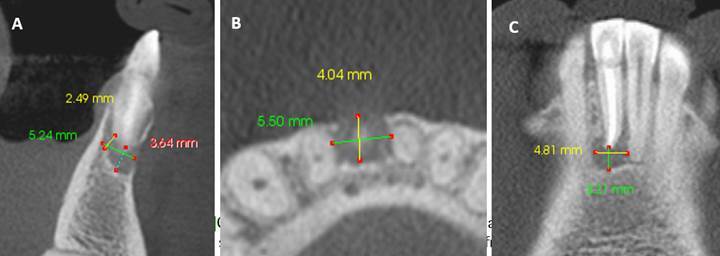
CBCT - PAI 4 +D (destruction), which was the most frequent lesion
